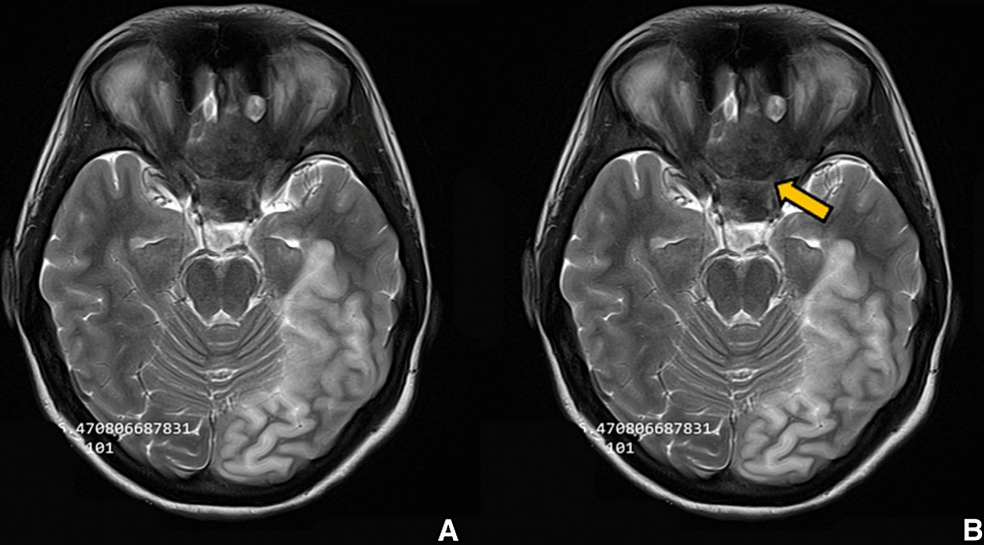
Preoperative imaging for patient 2. Plain T2-weighted axial image showing sellar, suprasellar lesion. (A) Without ROI and (B) with ROI.
